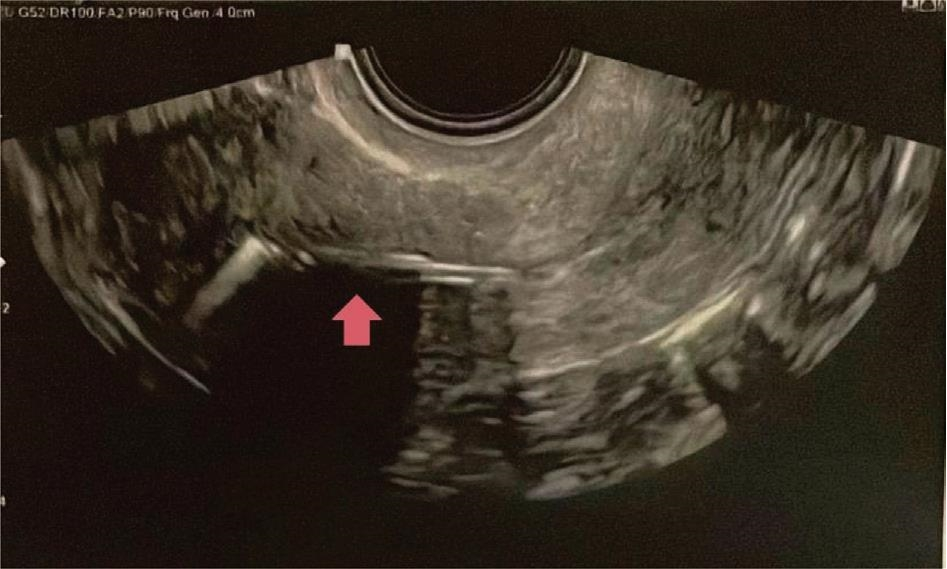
Postinsertion ultrasound image of the IUS. The device is correctly positioned.
